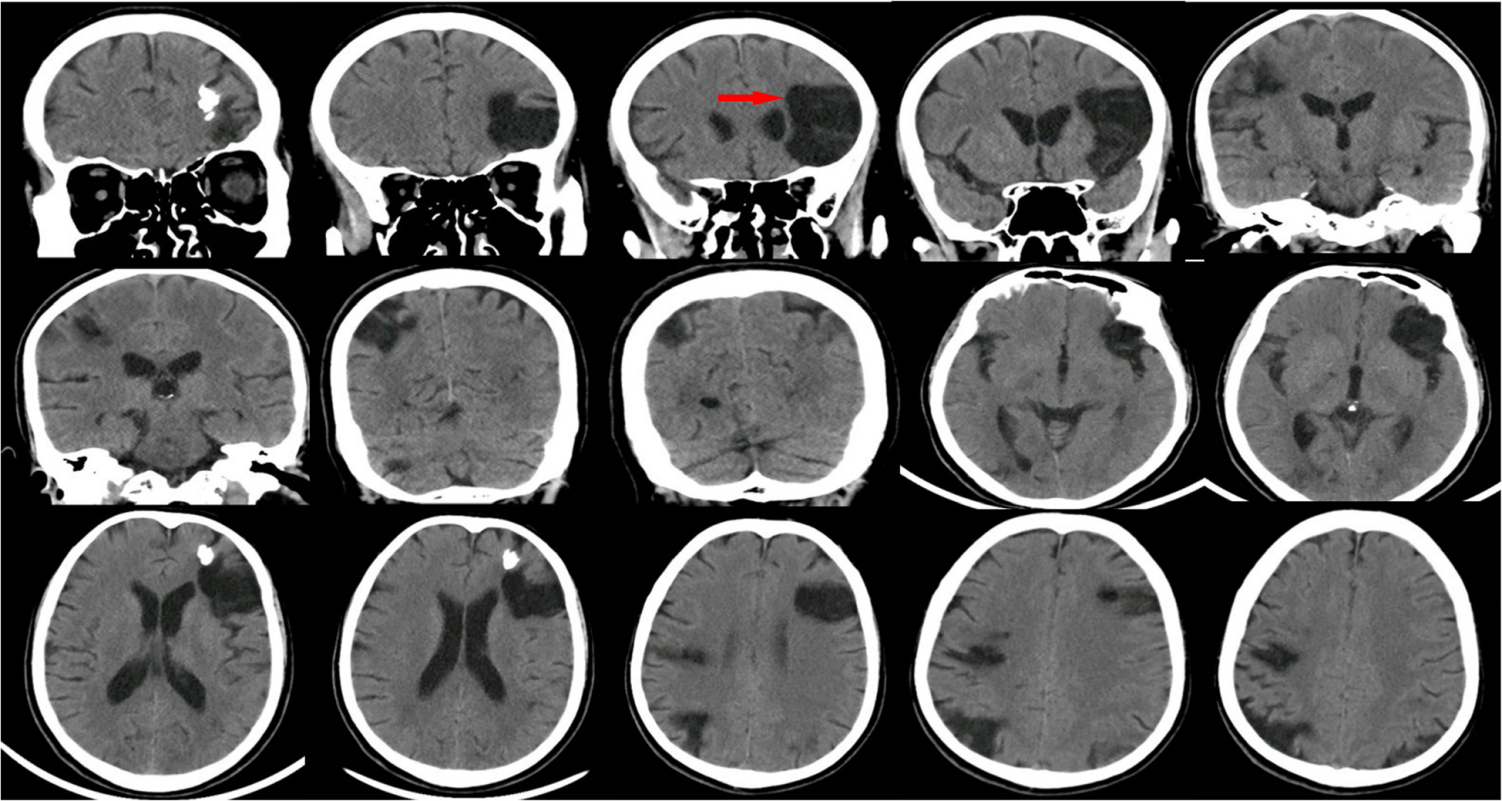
Computed tomography (CT) indicated cerebral infarctions (red arrow)
